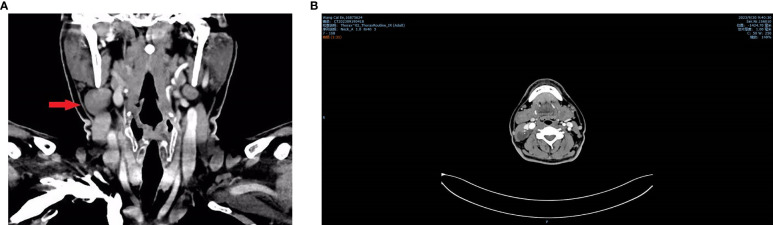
Neck CT plain scan + enhancement shows a solid-cystic mass with calcification in the right side of the neck. (A) Coronal view. (B) Horizontal view.
